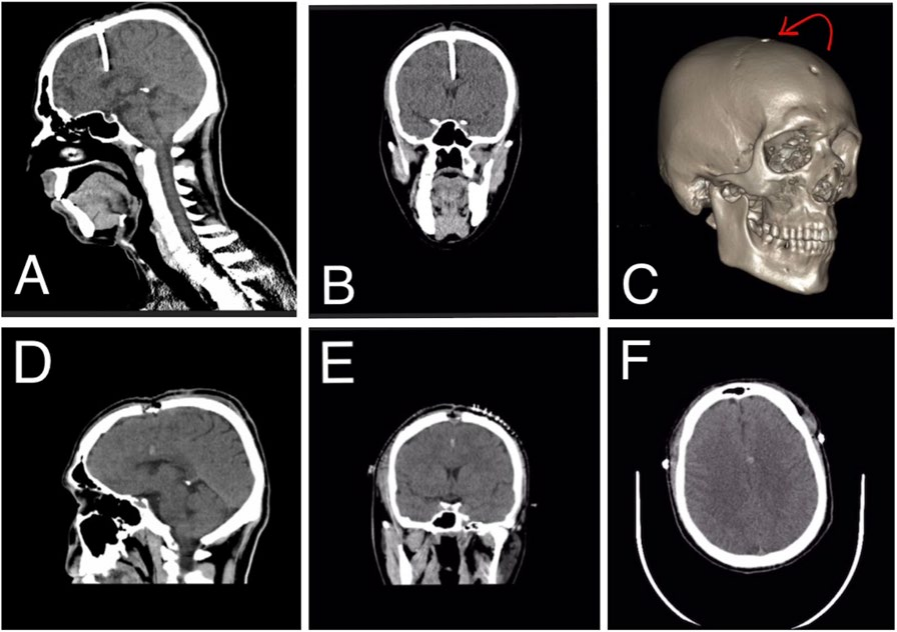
A Preoperative sagittal view of CT brain plain showing single nail projecting at the level of the junction between anterior 1/3rd and middle 1/3rd of superior sagittal sinus, no associated hemorrhage or signs of raised ICP. B Preoperative coronal view of CT brain plain showing midline penetrating object just above frontal horn of lateral ventricle. C Computed tomography three-dimensional reconstruction of skull showing foreign body entry (red arrow). D Postoperative CT brain plain sagittal view showing craniectomy, and small hemorrhagic point, while no remnants of the foreign body were seen. E Postoperative coronal CT brain plain. F Post-operative axial CT brain plain
